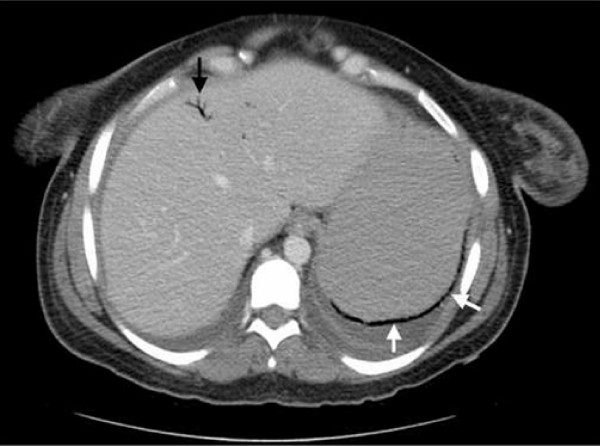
CT of the abdomen showing air in the stomach wall and portal venous system. Black arrow: Portal venous air. White arrows: Air in the stomach wall.
